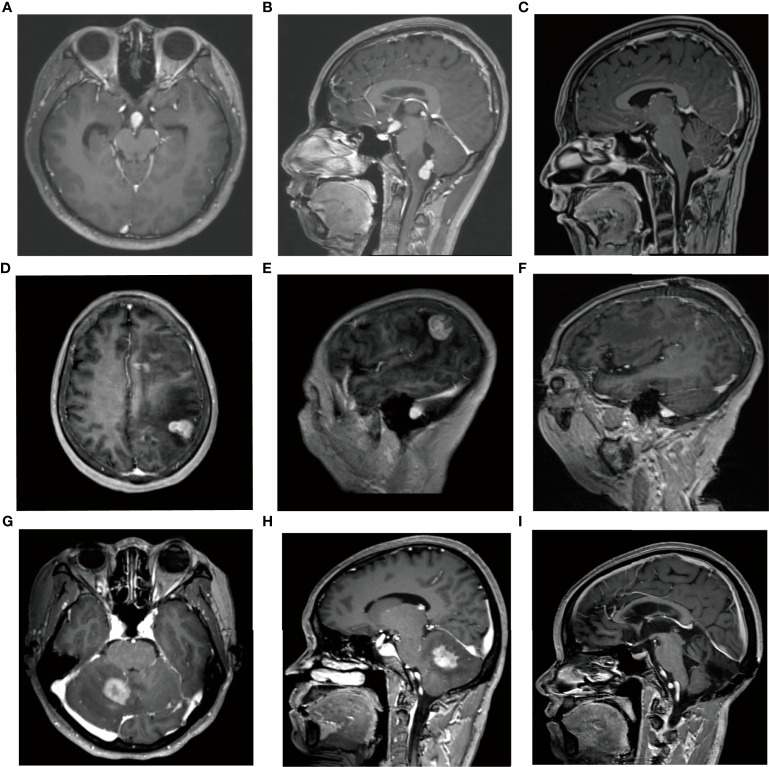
Case 1, male, 16 years old. (A, B) MRI-enhanced images showed homogeneous enhancement of tumors in the dorsal side of the brainstem, around the fourth ventricle, and in the pineal region. (C) Postoperative MRI showed gross total resection of brainstem lesions. (D, E) Case 2, female, 52 years old. Sagittal-enhanced images showed significantly enhanced tumor masses in the left parietal lobe. (F) The tumor was totally resected. (G, H) Case 38, female, 48 years old, right cerebellar hemisphere aera displayed homogeneous enhancement. (I) Postoperative MRI revealed surgery combined with radiotherapy and chemotherapy obtained a good therapeutic effect.
